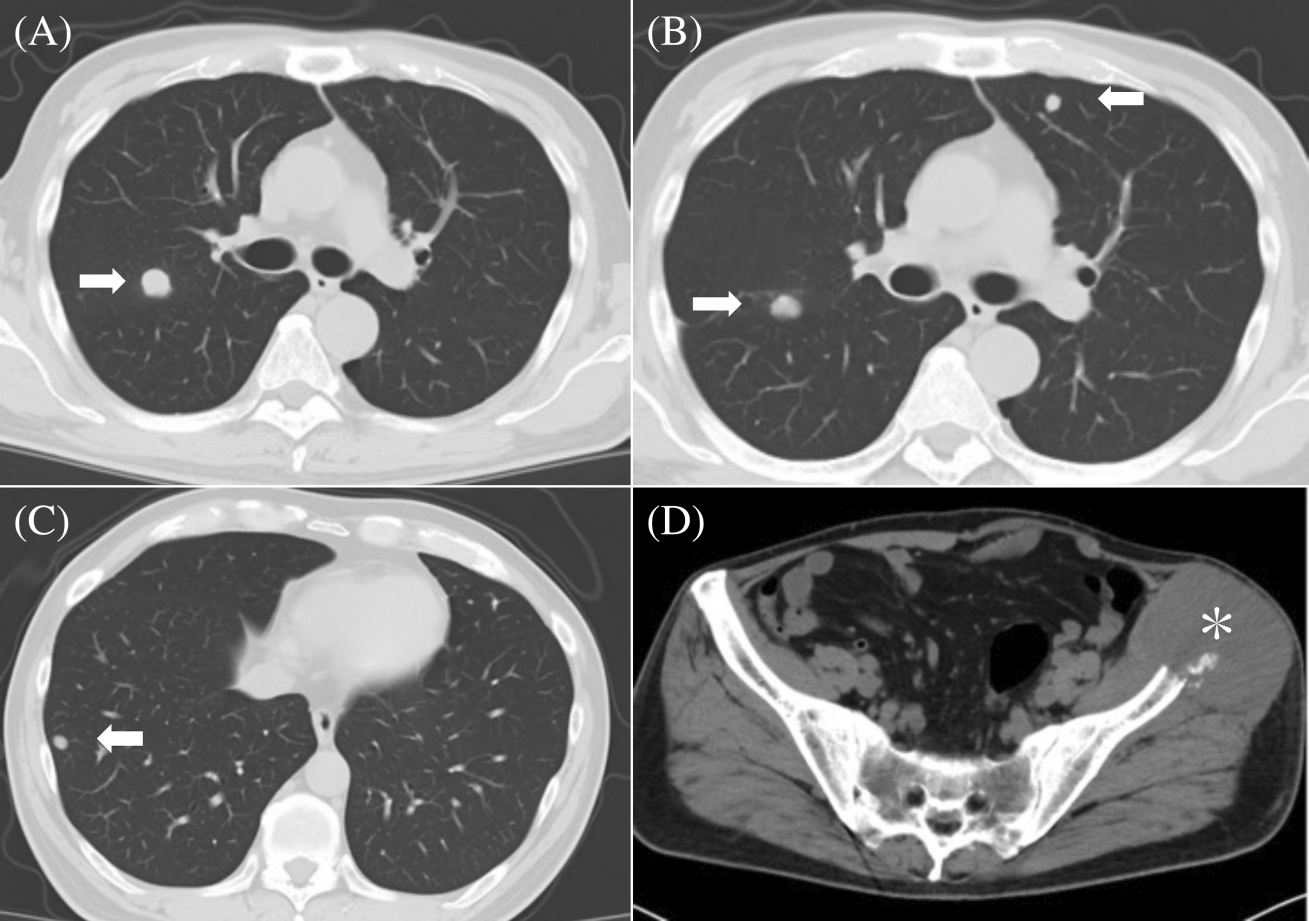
Computed tomography imaging after five courses of nivolumab administration. (A–C) Progression of multiple lung metastases (white arrows) and (D) left iliac bone metastases (asterisks) are observed.
